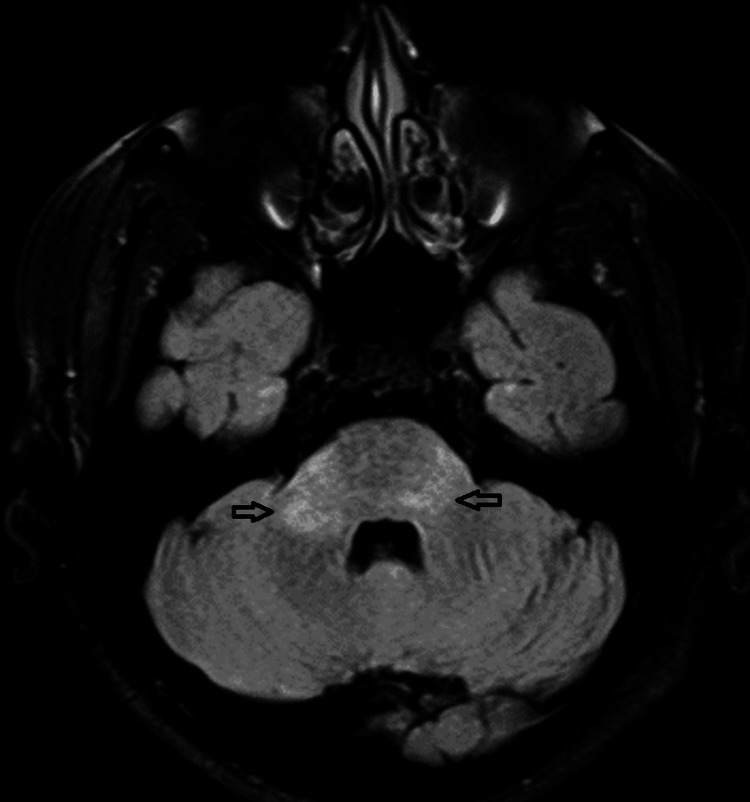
Hyperintense areas are seen in the pons and both middle cerebellar peduncles (RT >LT) on FLAIR images FLAIR - fluid attenuated inversion recovery, RT - right, LT - left
